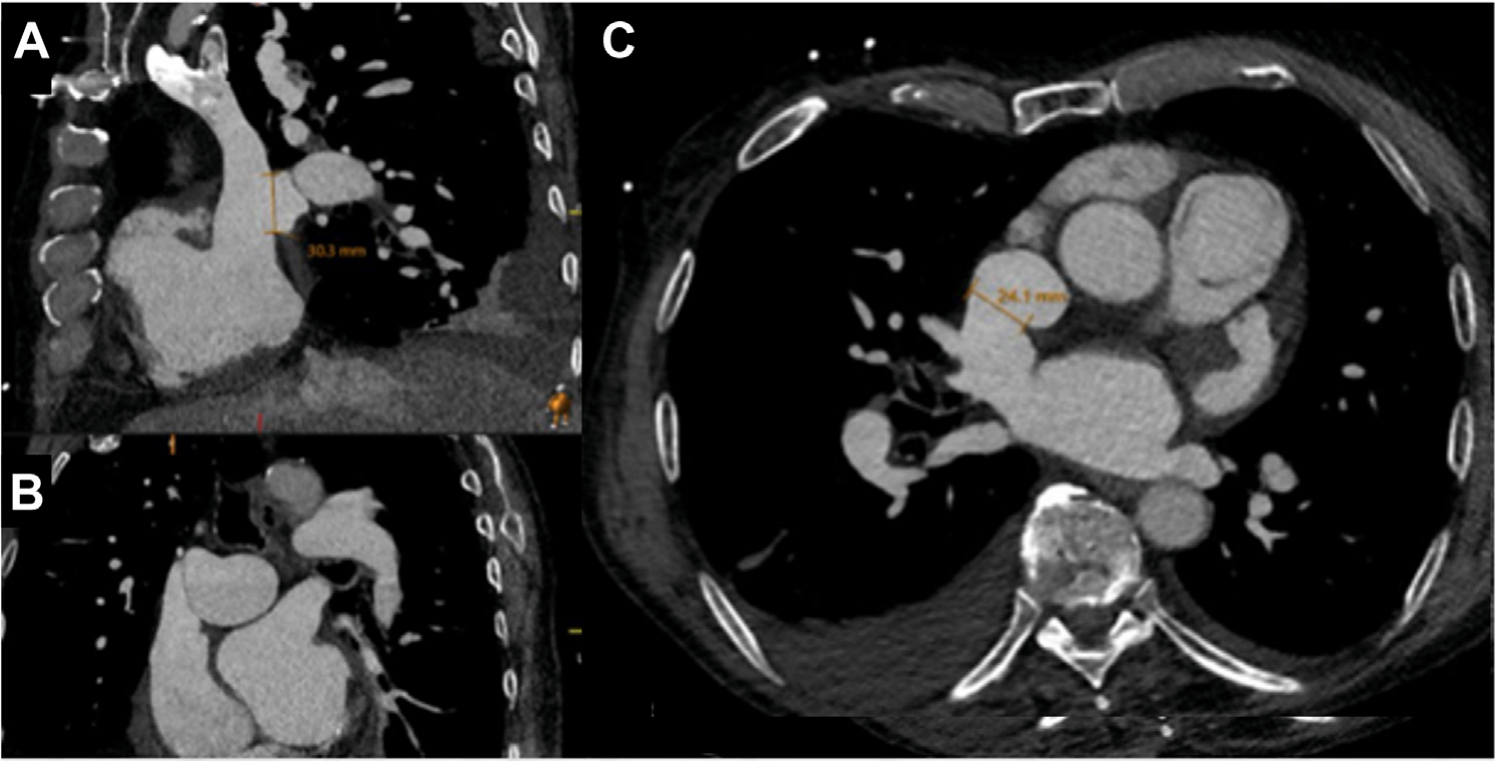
Superior Sinus Venosus Atrial Septal Defect With Partial Anomalous Pulmonary Venous Return Multiplanar (A) sagittal, (B) coronal, and (C) axial computed tomography images of the sinus venosus atrial septal defect with partial anomalous pulmonary venous return of the right upper and middle pulmonary veins.
